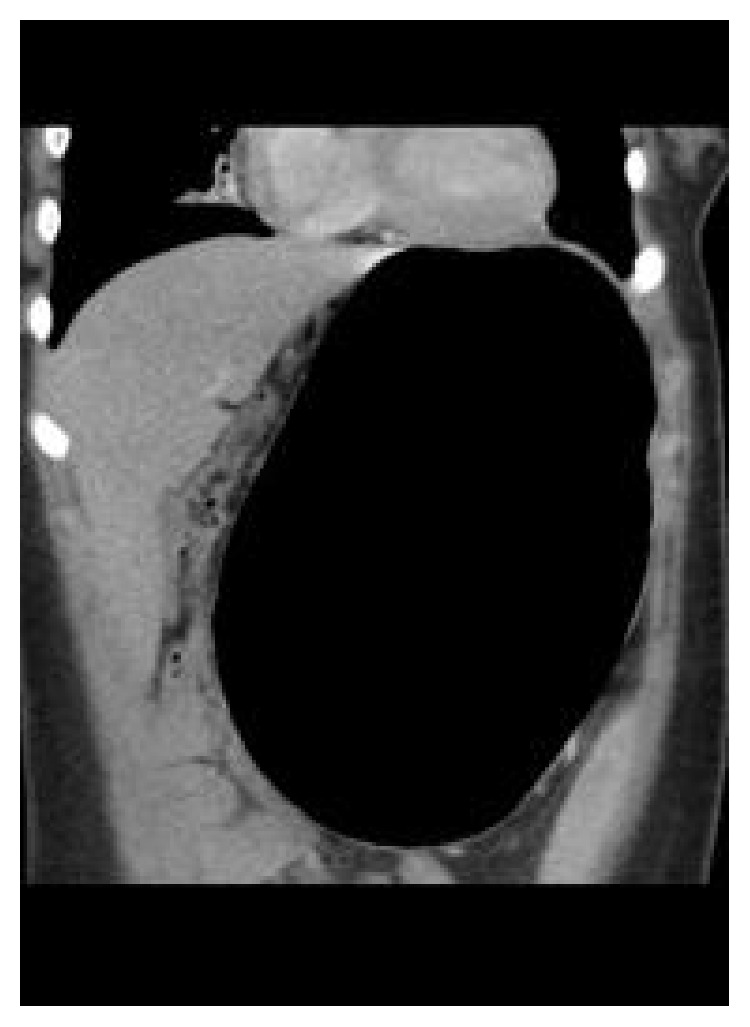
The coronal computed tomography scan of the abdomen showing persistent gastric dilatation.
